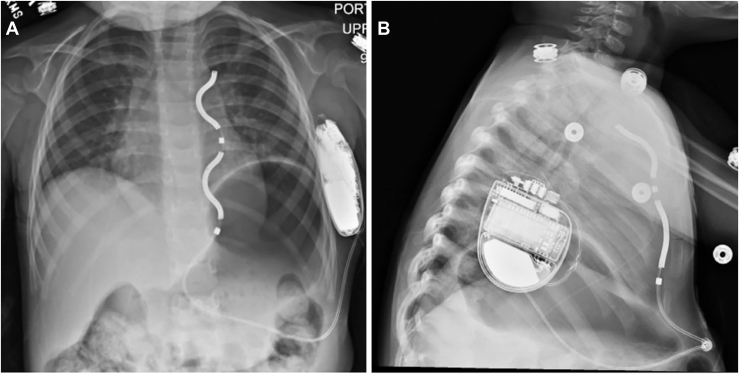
Radiographic confirmation of device placement. (A) Posteroanterior and (B) lateral chest roentgenograms demonstrating appropriate position of the lead and generator.
